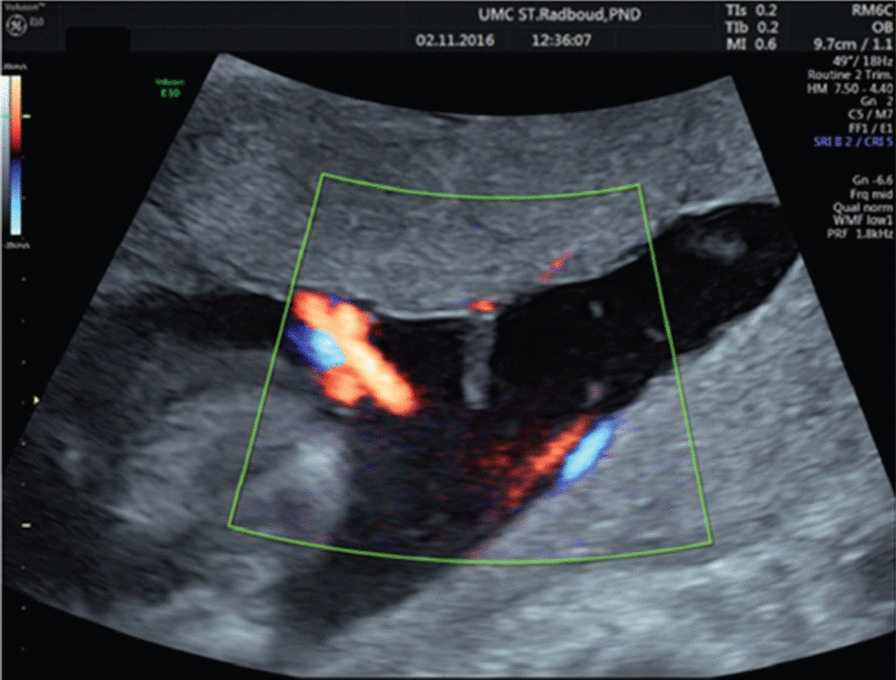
Postoperative ultrasonographic image of umbilical cord at placental insertion after cord transection
